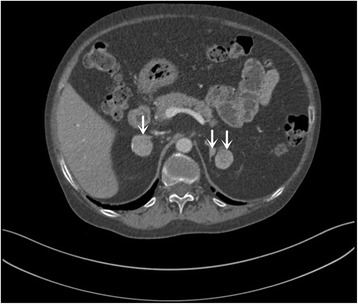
Adrenal computed tomography (CT) of the patient. Adrenal CT showed a right adrenal nodule with a diameter of 2.5 cm, and two left nodules with diameters of 2.3 cm and 0.6 cm, respectively (arrows)
